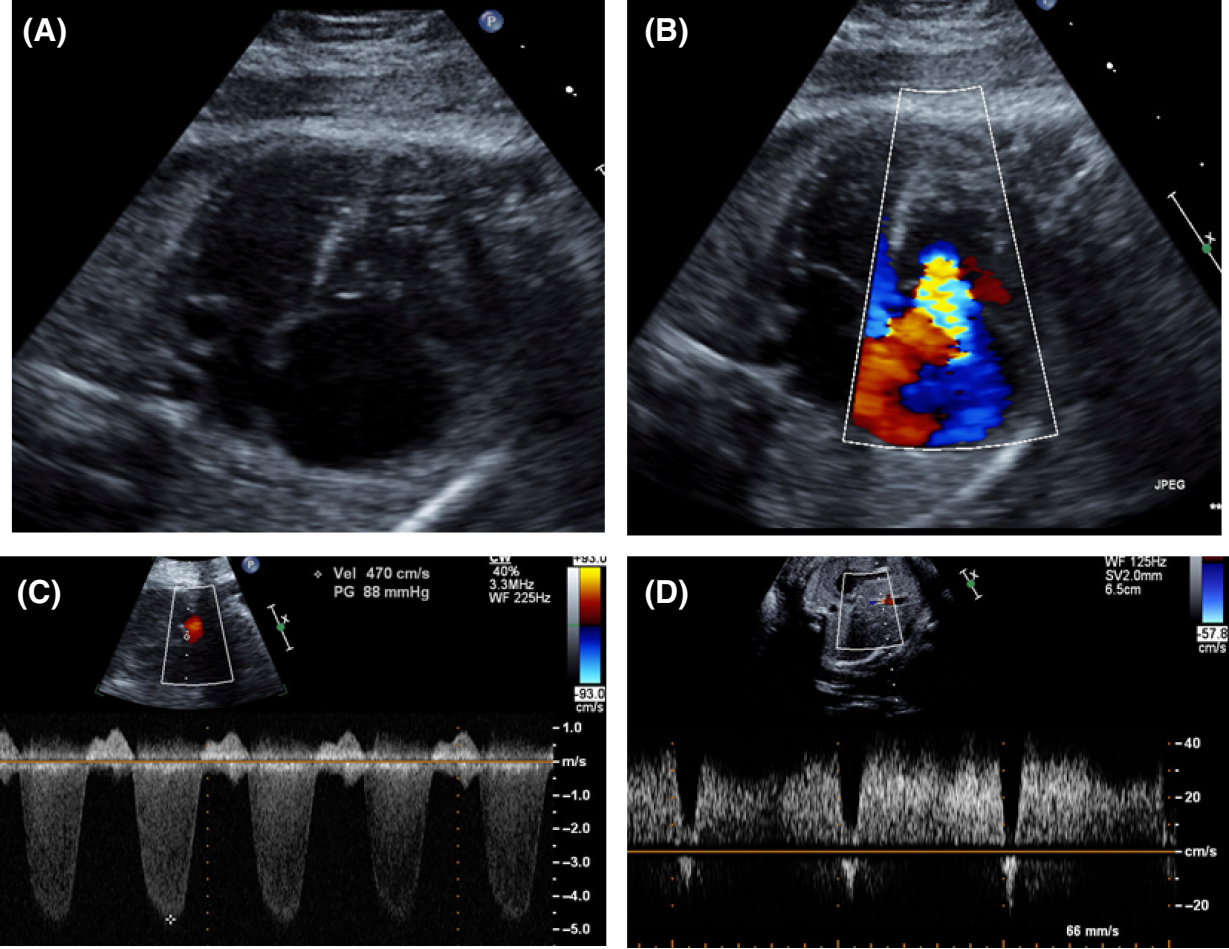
Fetal echocardiogram at 22‐week gestation. (A) Four‐chamber view showing severe right atrial dilation, dilated tricsupid valve, and right ventricular hypertrophy. (B) Four‐chamber view with color flow on the tricuspid valve showing severe tricuspid regurgitation. (C) Continuous‐wave Doppler of the tricuspid regurgitation jet showing severe right ventricular hypertension. Estimated right ventricular pressure is 88 mm Hg plus the right atrial v‐wave. (D) Doppler flow profile of the ductus venosus showing a‐wave flow reversal.
